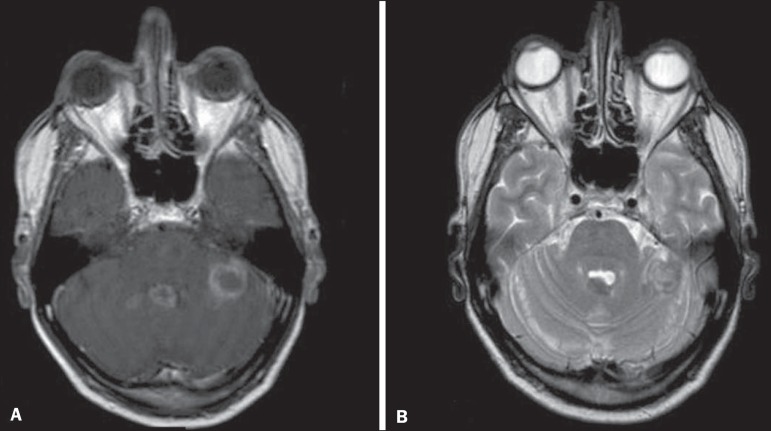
Paracoccidioidomycosis. Gadolinium contrast-enhanced T1-weighted MRI
